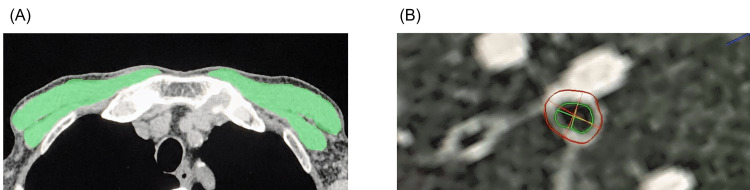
Representative cross-sectional computed tomography images. (A) The pectoralis muscle area is shown in green. (B) Lumen area (green) and wall area (between green and red) of the segmental right apical (third-generation of the segmental right apical).
